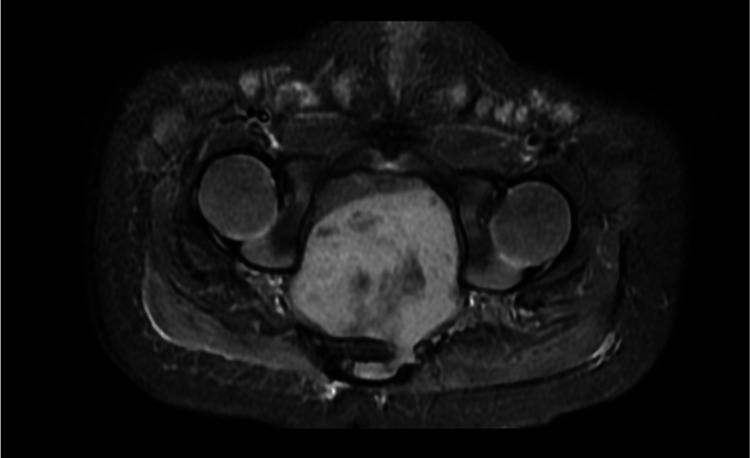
The axial image of the MRI scan revealed a large heterogeneous presacral mass extending to the spinal canal through the dilated neural exit foramina. No evidence of bone erosions.
